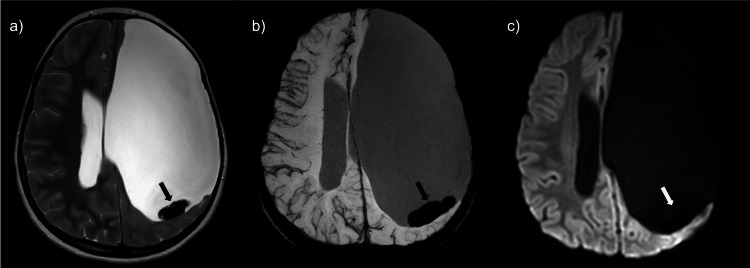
MR brain images demonstrate a significant decrease in the size of the lesion when compared to the prior CT. (a) Axial T2WI demonstrates a dependent T2 hypointense lesion (black arrow). (b) SWI shows blooming artifact consistent with blood products (black arrow). (c) DWI demonstrates no diffusion restriction (white arrow).
